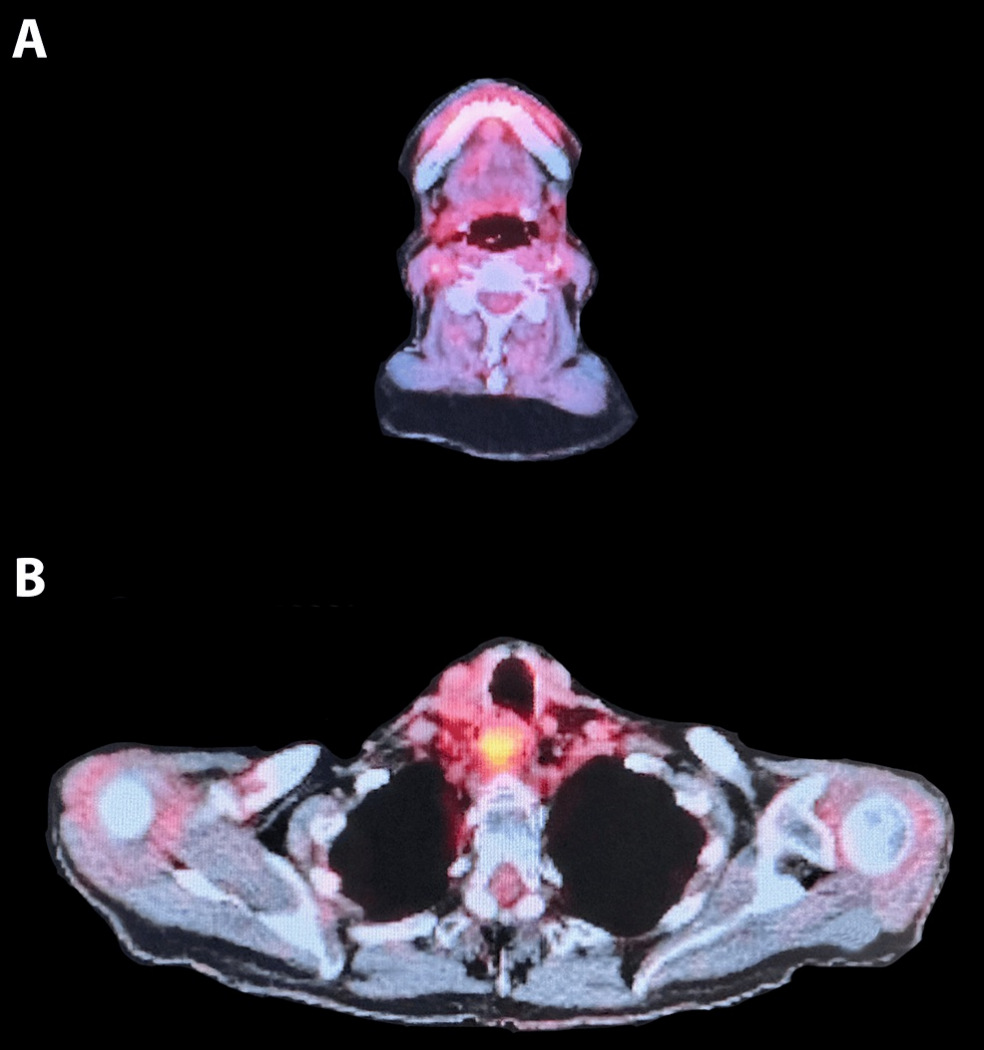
Post-chemotherapy and bone marrow transplant imaging: no definitive PET/CT evidence of malignancy. Metabolically active soft tissue fullness in the proximal esophagus is stable compared to the previous study. A: Axial image of the previously metabolic level II node. B: Axial image of previous metabolic esophageal mass.
